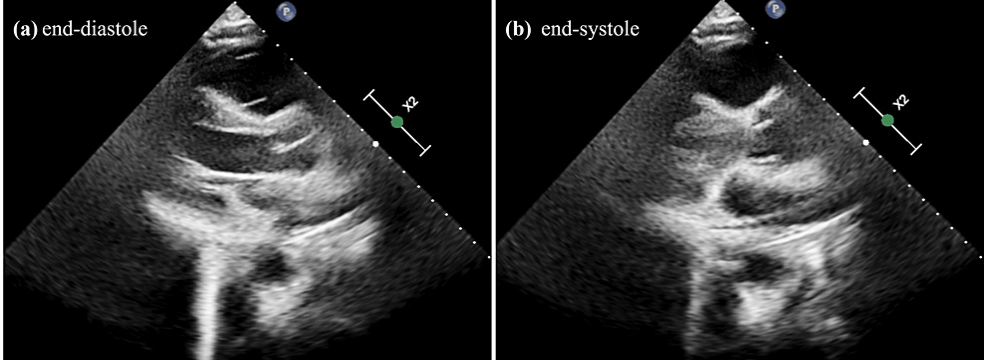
Transthoracic echocardiogram one month after the discontinuation of bicalutamide Transthoracic echocardiogram parasternal long axis view one month after the discontinuation of bicalutamide: (a) end-diastole and (b) end-systole. The left ventricular ejection fraction returned to the baseline value of 60%
